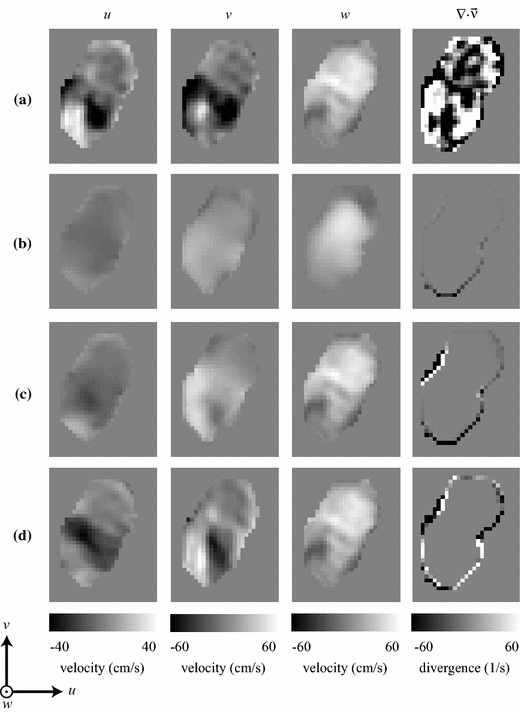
Velocity maps for the individual velocity components (*u*, *v*, and *w*) and divergence map of the velocity field ($$\vec {\nu }$$), for a slice perpendicular to the carotid bifurcation of a healthy volunteer: a PC-MRI; b CFD; c CFD guided by PC-MRI data corresponding to the main velocity component ($$w_{\mathrm {mri}}$$); and d CFD guided by PC-MRI data corresponding to all three velocity components ($$u_{\mathrm {mri}}$$, $$v_{\mathrm {mri}}$$, and $$w_{\mathrm {mri}}$$)
